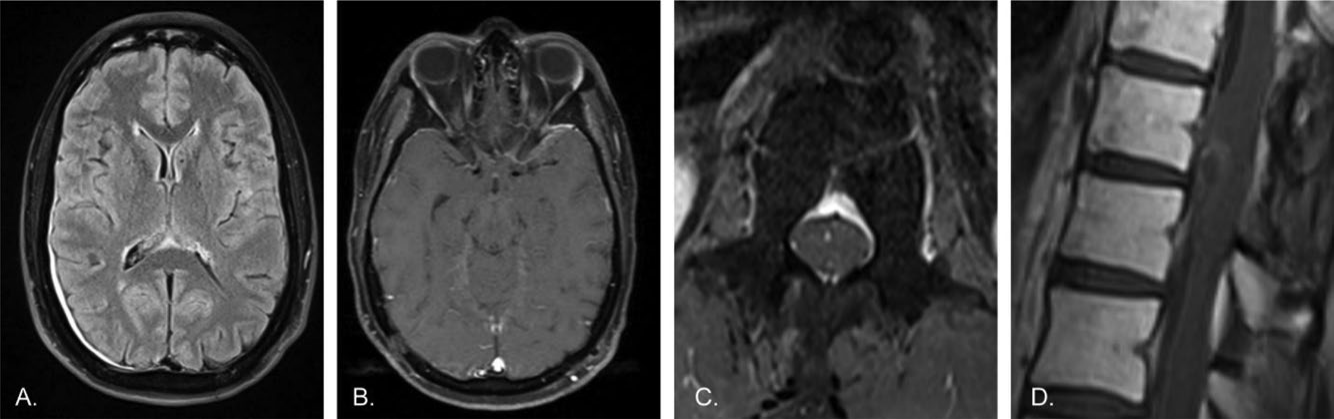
Restaging MRI 3 months following initiation of C1D1 treatment with tovorafenib. A) Axial T2-FLAIR image of the brain shows persistent but markedly reduced leptomeningeal hyperintensity compared to prior. Persistent dural thickening is again likely post-surgical in nature. B) Axial post-contrast T1 image of the brain demonstrates markedly decreased leptomeningeal enhancement, with no clear residual enhancement beyond what may represent normal background. C) Axial post-contrast T1 image of the lumbar spine shows no discernible enhancement of the cauda equina, now appearing essentially normal. D) Sagittal post-contrast T1 image of the lower thoracic spine shows a significant reduction in cord surface enhancement, with only faint residual enhancement of the previously noted mass-like lesion along the ventral cord. Overall, findings are significantly improved compared to all previous time-points, including the initial presentation.
